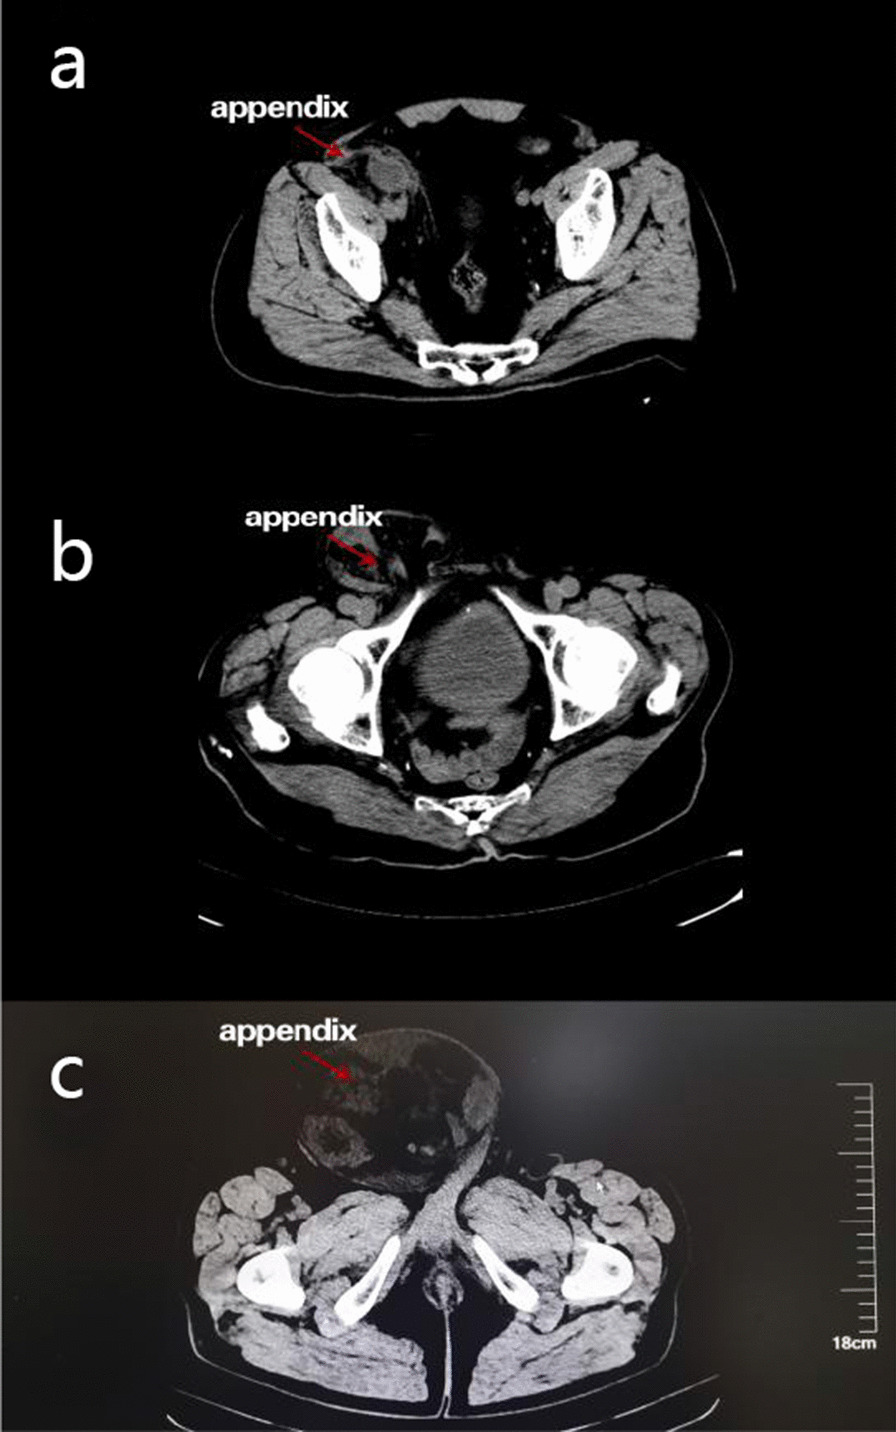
CT images of patients with Amyand’s hernia. a Preoperative CT images of appendicitis in Case 1. b Preoperative CT images indicated that the appendix was located in the hernia sac in Case 5. c Preoperative CT images indicated that the appendix was located in the hernia sac in Case 6
